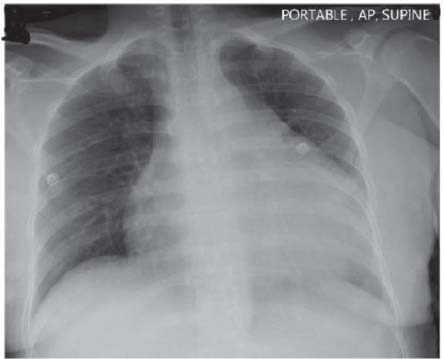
Initial Chest x-ray showing increased cardiothoracic ratio.
